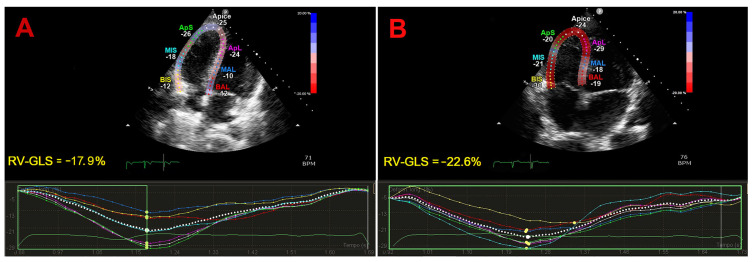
Representative examples of RV-GLS magnitude obtained by STE in a patient with mild-to-moderate IPF (A) and in a healthy control without IPF (B). The dotted line indicates the average RV-GLS, determined by averaging strain values of the segments from the free lateral wall and the segments from the interventricular septal wall. GLS, global longitudinal strain; IPF, idiopathic pulmonary fibrosis; RV, right ventricular; STE, speckle tracking echocardiography.
